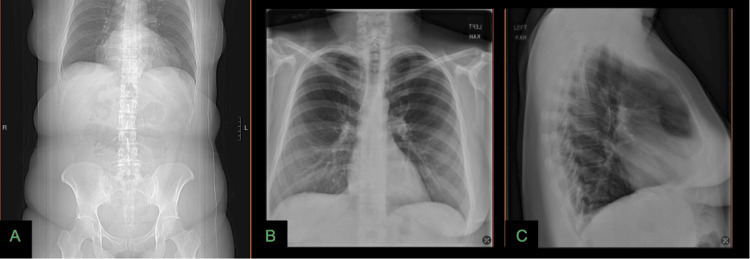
Preoperative and postoperative radiographic imaging of a Larrey hernia. (A) Preoperative CT scout image demonstrating an opacity overlying the mediastinum with soft tissue and air consistent with herniation of a hollow viscus. (B-C) Anteroposterior and lateral chest X-rays obtained five months after Larrey hernia repair, showing normal contour of the hemidiaphragm; no intrathoracic bowel loops; absence of retrosternal masses; clear lung fields; and no pleural effusion.
